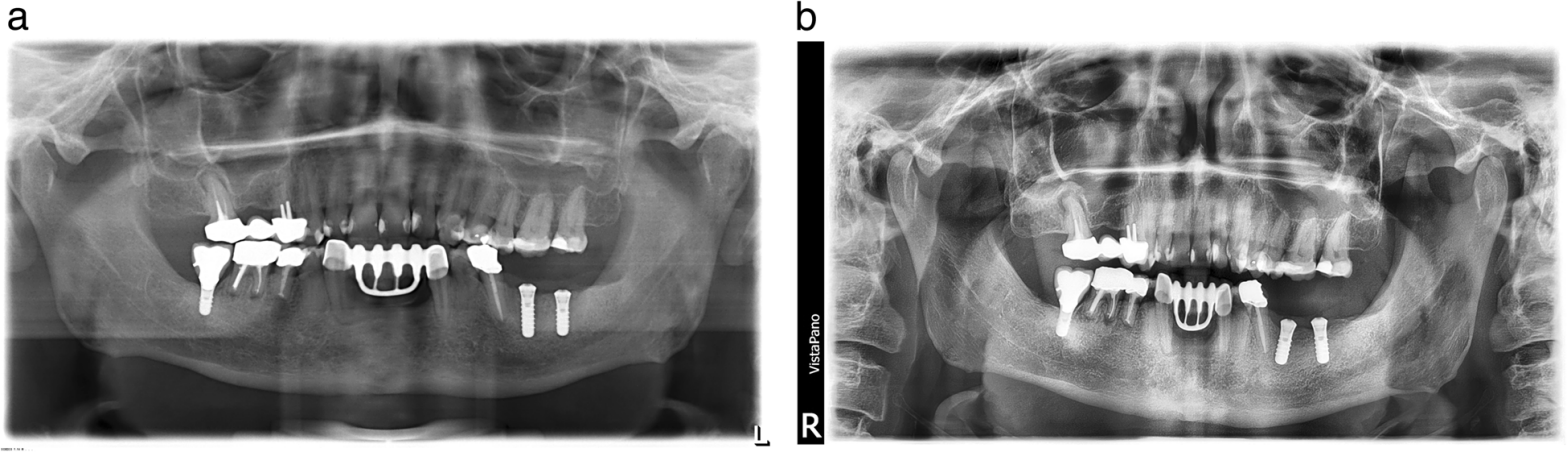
a Patient 2. Postoperative orthopantomogram one day after implant placement. b Patient 2. Postoperative orthopantomogram after second Implant placement
